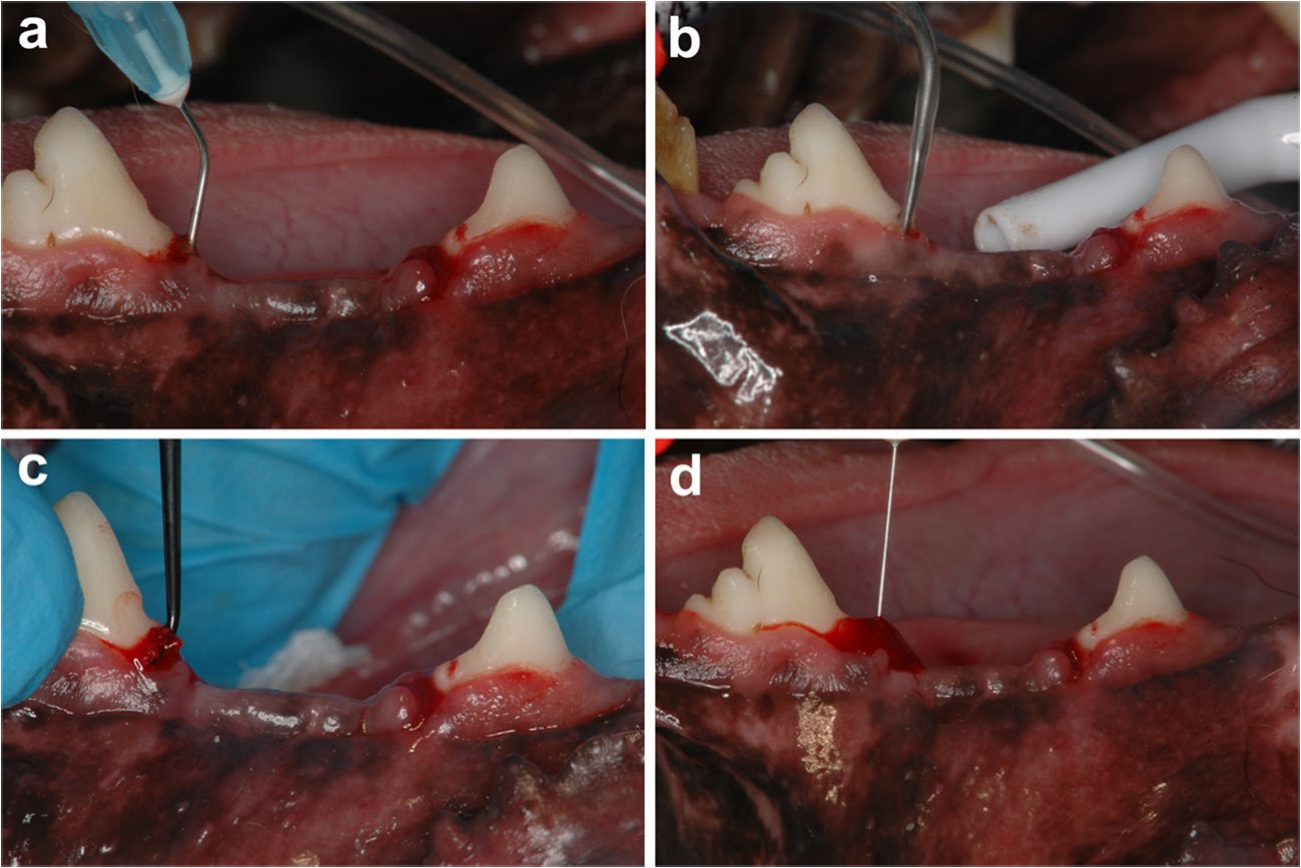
a Application of sodium hypochlorite/amino acid gel to the periodontal pocket. SRP performed using an ultrasonic device with an ultrasonic tip (b) and hand instruments (c). d Application of a xHyA gel to the periodontal pocket
